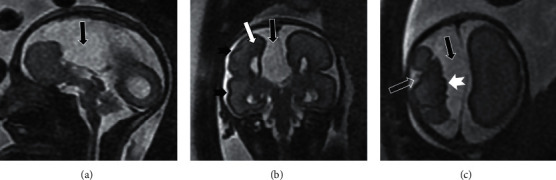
Complete agenesis of corpus callosum. Gestational age: 27 weeks. (a) Sagittal SSFSE T2. (b) Coronal SSFSE T2. (c) Axial SSFSE T2. Complete agenesis of corpus callosum with right interhemispherical cyst with mass effect (solid black arrow) is shown. The right cerebral hemisphere is smaller in size, and malformation of cortical development is evident, with multiple anomalous sulci, bumpy cortical surface (solid black arrowhead), and cortical invaginations (empty white arrow). Regions of associated polymicrogyria (solid white arrowhead) and heterotopias bulging on the ventricle wall are depicted (solid white arrow). Fetal MRI provides useful information for perinatal management and prognosis over fetal ultrasound by detecting anomalies of the cerebral cortex and heterotopies not visible in the fetal ultrasound.
